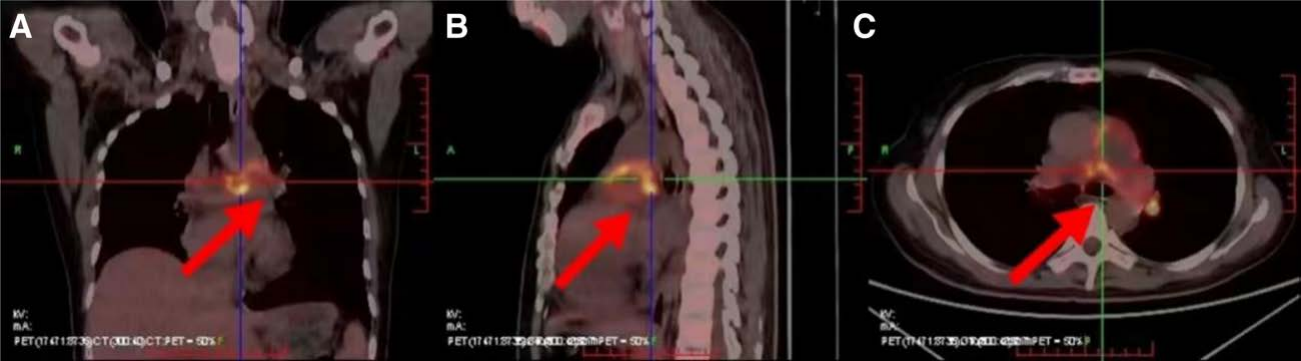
PET-CT whole-body imaging in coronal, sagittal, and cross-sectional views shows limited nodular metabolic increase from the main pulmonary artery trunk to the bifurcation of the right and left branches A: Coronal; B: Sagittal; C: Cross-sectional views. PET-CT = positron emission computed tomography.
